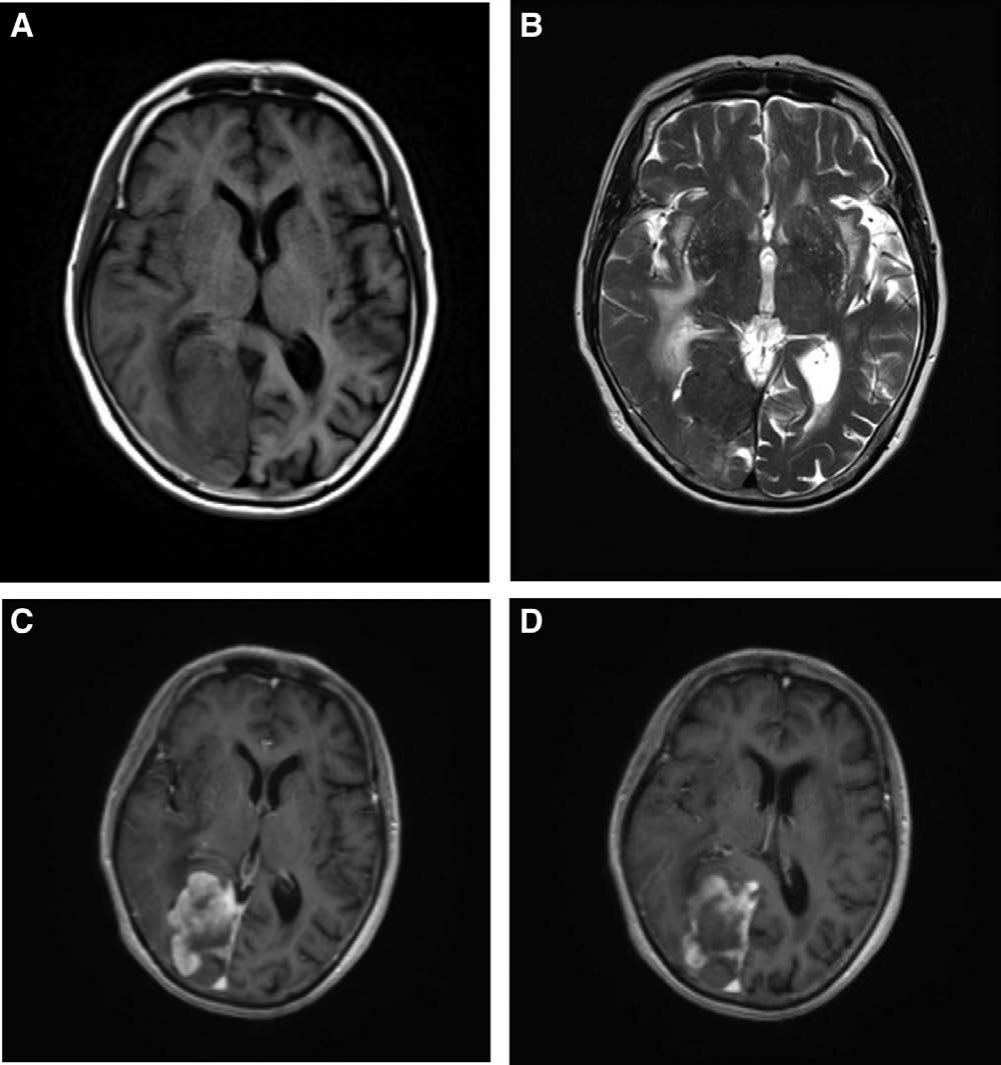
Cranial MRI in 2022: an irregular lobulated mass with moderate peritumoral edema in the right occipital region with a maximum diameter of 5.4 cm; heterogeneous enhancement after contrast-enhanced scan (C and D). MRI = magnetic resonance imaging.
